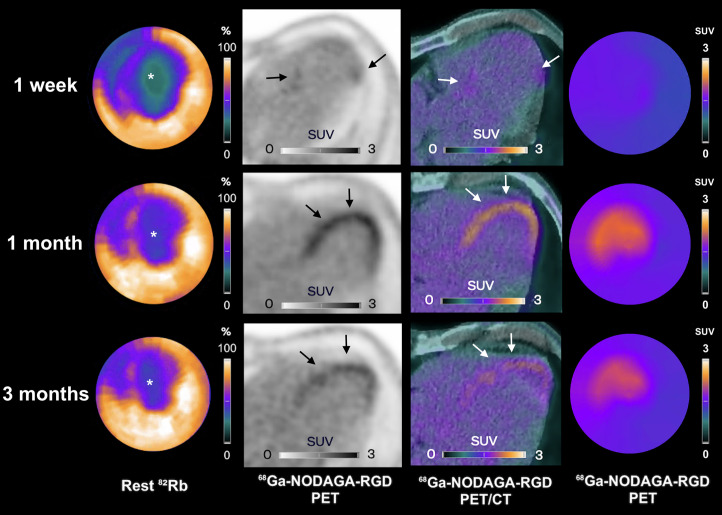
Participant example with increase in α_v_β_3_ integrin expression levels (arrows) throughout follow-up within infarcted myocardium (asterisks). Shown are rest ^82^Rb, ^68^Ga-NODAGA-RGD PET, and ^68^Ga-NODAGA-RGD PET/CT images at 1 wk (first row), 1 mo (second row), and 3 mo (third row) after STEMI. ^68^Ga-NODAGA-RGD uptake started in border zone at 1 mo and subsequently extended into necrotic infarct core at both 1 and 3 mo.
